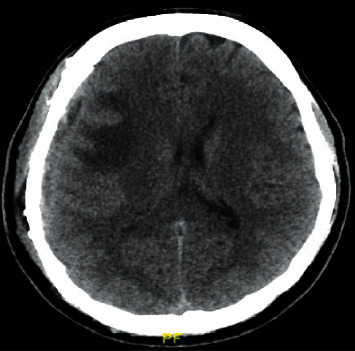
Noncontrast axial CT head showing a new area of diminished attenuation in the right frontal lobe suggestive of vasogenic edema.
